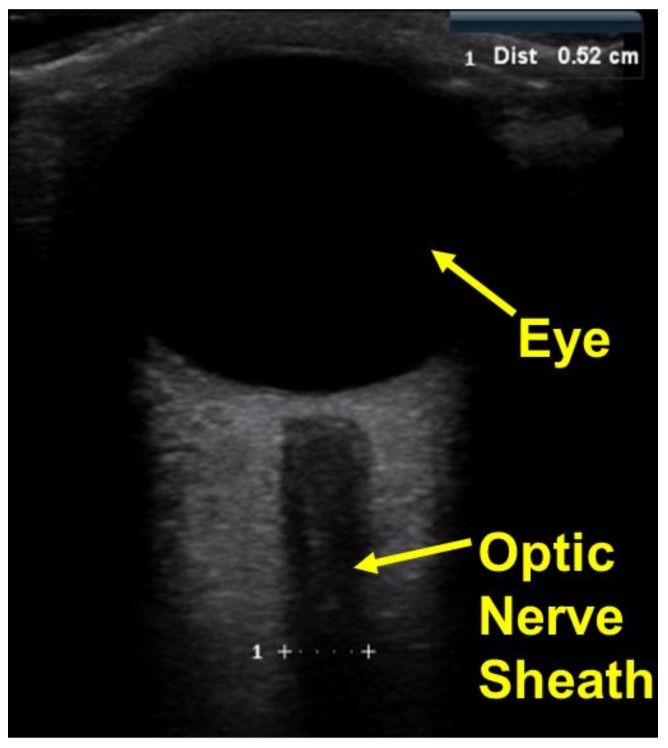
Optic nerve sheath diameter data was obtained from ultrasound imaging of the eye and used to calculate intracranial pressure (ICP).
